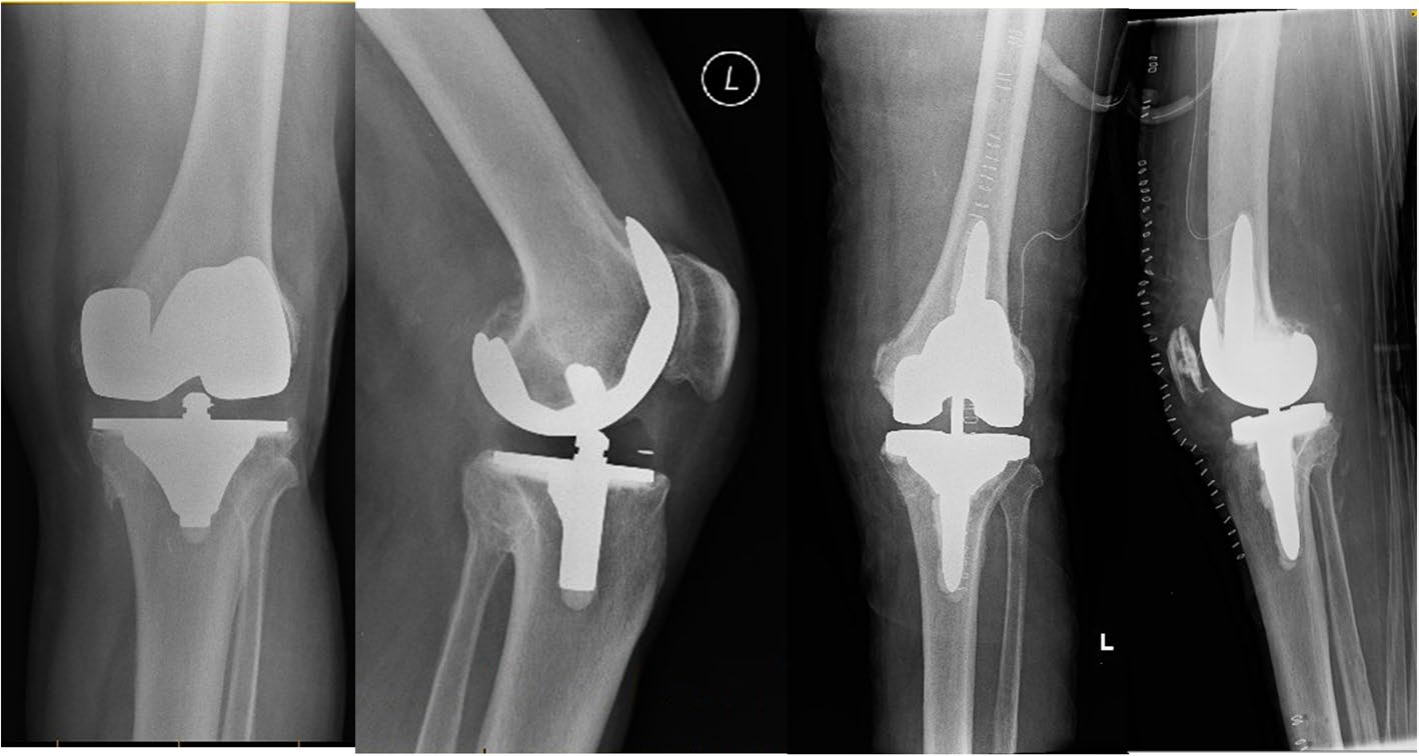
Pre- and postoperative radiographs of patient for preoperative planning in Fig. 8
